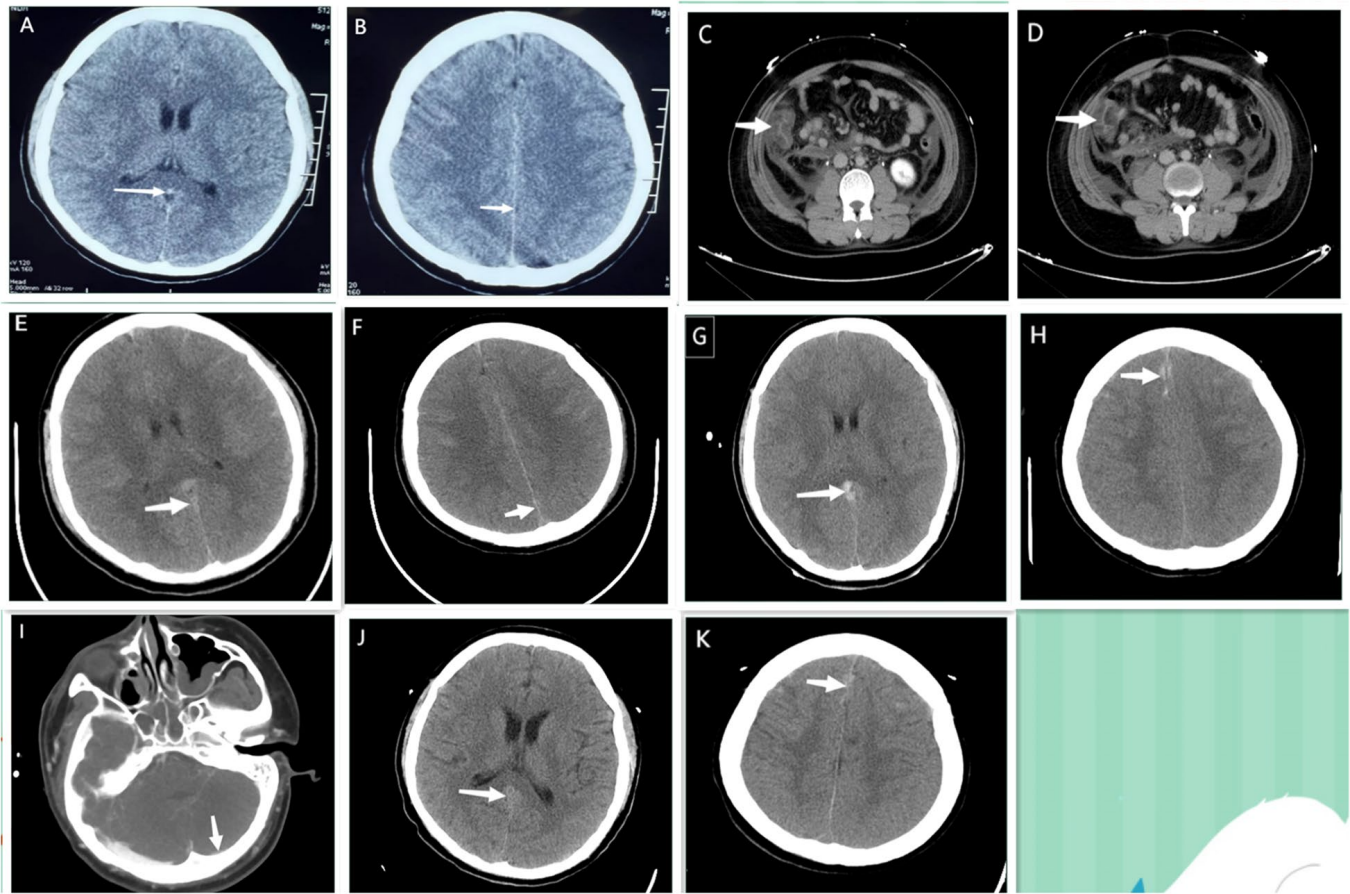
Radiographic findings of the patient. A-B showed cerebral edema and subarachnoid hemorrhage by cranial CT in local hospital; C-D showed local edema and thickening of the ascending colon wall with peritoneal thickening by abdominal CT plain scans in our hospital; E–F showed aggravated cerebral edema and subarachnoid hemorrhage by CT One day later; G-H showed significantly aggravated cerebral edema and multiple subarachnoid hemorrhages by CT After 12 days; I showed fine contrast in the left transverse sinus, sigmoid sinus and the opposite side of the internal jugular vein, and less uniform local density of transverse sinusAfter 12 days; J ~ K showed alleviated cerebral edema and subarachnoid hemorrhage after 2 weeks
